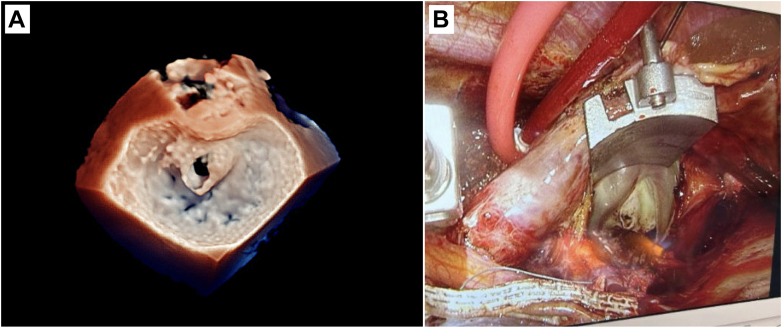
Anterior Mitral Valve Leaflet Perforation Before Repair (A) Three-dimensional echocardiography and (B) intraoperative photograph of the anterior mitral valve leaflet perforation before repair.
